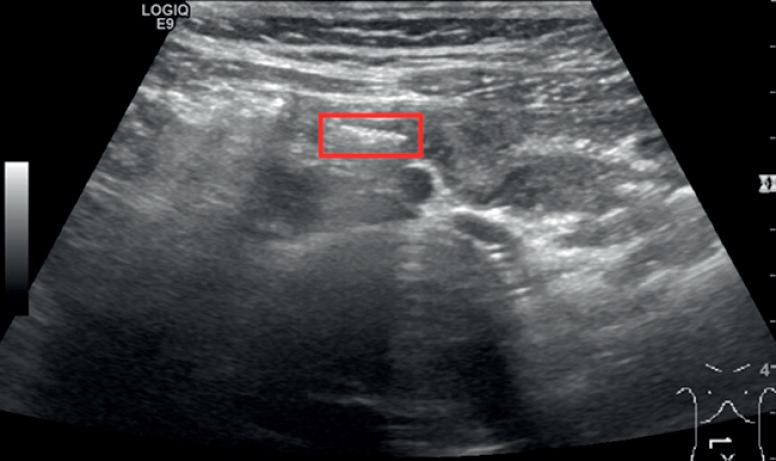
Ultrasound imaging of the appendix demonstrated the intraluminal position of the screw (red rectangle).
